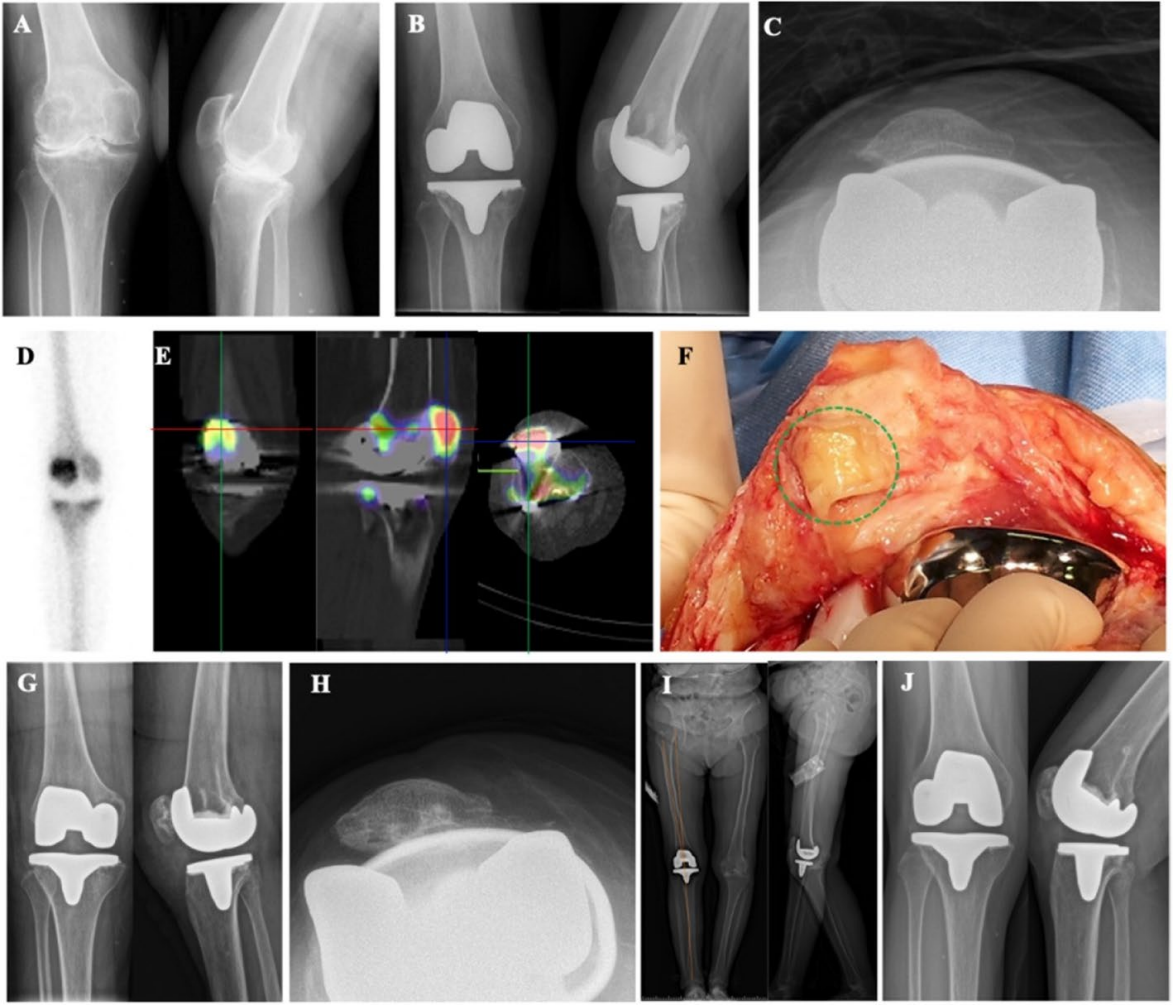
Another instance of SPR. A Standing anteroposterior and lateral radiographs of the right knee exhibit narrowing of the medial compartment, indicative of osteoarthritic (OA) changes. B Post-TKA anteroposterior and lateral radiographs of the right knee demonstrate a prosthesis that is well-positioned with normal alignment. C Axial radiograph post-TKA confirms the alignment of the prosthetic components. D Triple-phase bone scan reveals radioactive concentration within the right knee joint. E SPECT/CT imaging identifies focal radionuclide concentration on the articular surface of the patella. F Intraoperative exploration discloses wear on the articular surface of the right patella accompanied by metal debris. G Standing anteroposterior and lateral radiographs following SPR. H Axial radiograph post-SPR. I Full-length functional radiograph post-SPR. J Radiographs at a seven-year follow-up post-SPR
